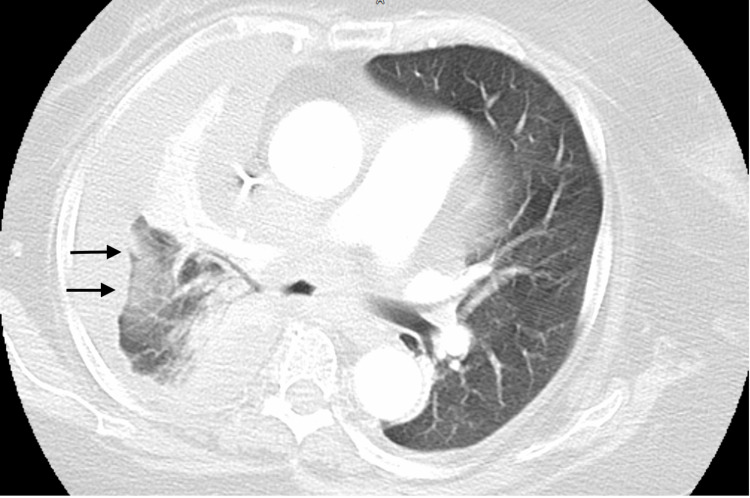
Follow up CT chest three months later showed compressive atelectasis of the right lung (arrows).
